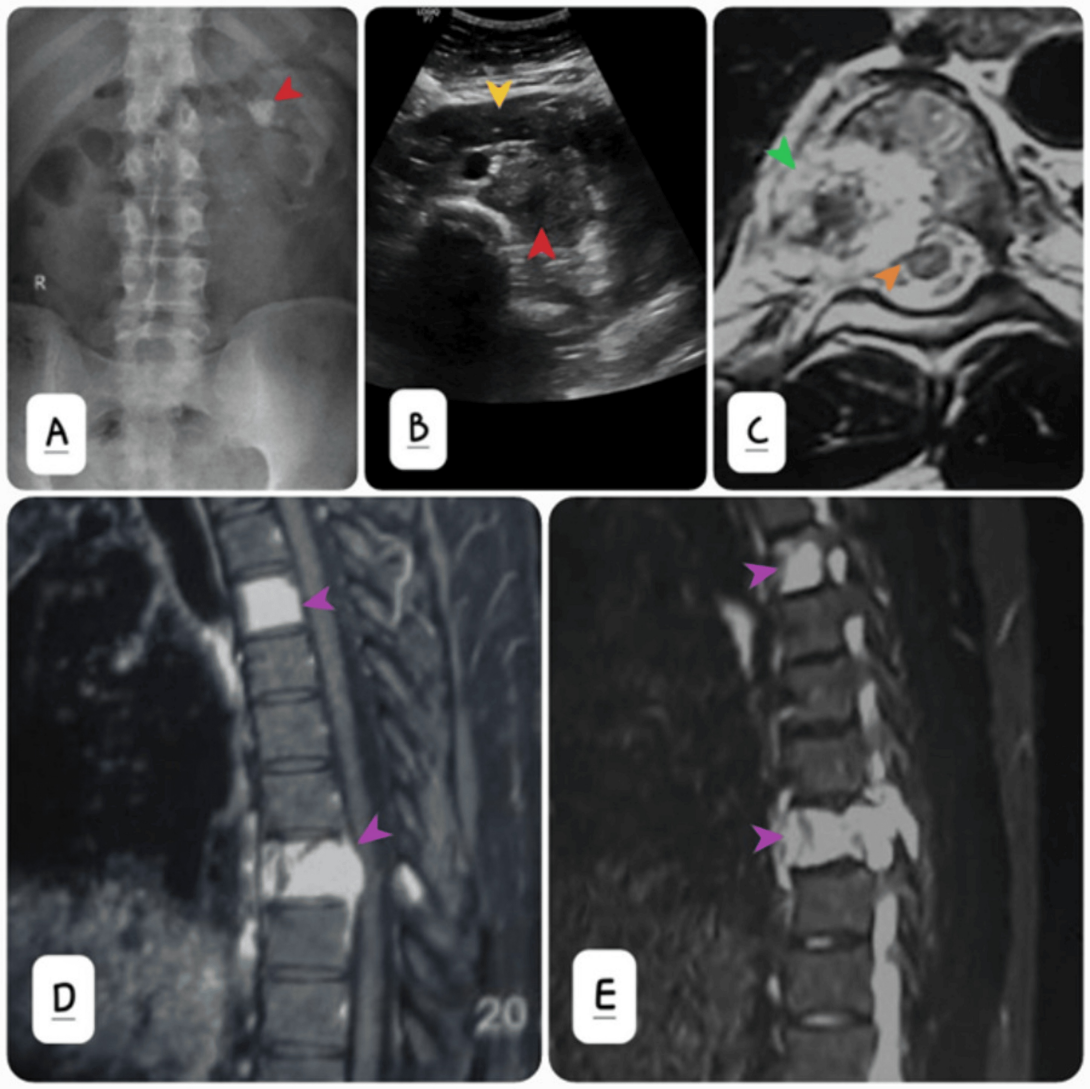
(A) Plain radiograph abdomen showing calcification in the mass at the left renal fossa (red arrow). (B) Transverse grey scale ultrasound showing horseshoe kidneys (yellow arrow) and left renal mass (red arrow). (C) Axial T2W MRI image showing osteolytic lesion (green arrow) in D8 vertebra with intraspinal extension of soft tissue component (orange arrow). (D) Post-contrast sagittal T1W and (E) Sagittal T2W MRI images showing vertebral metastasis (purple arrows).
